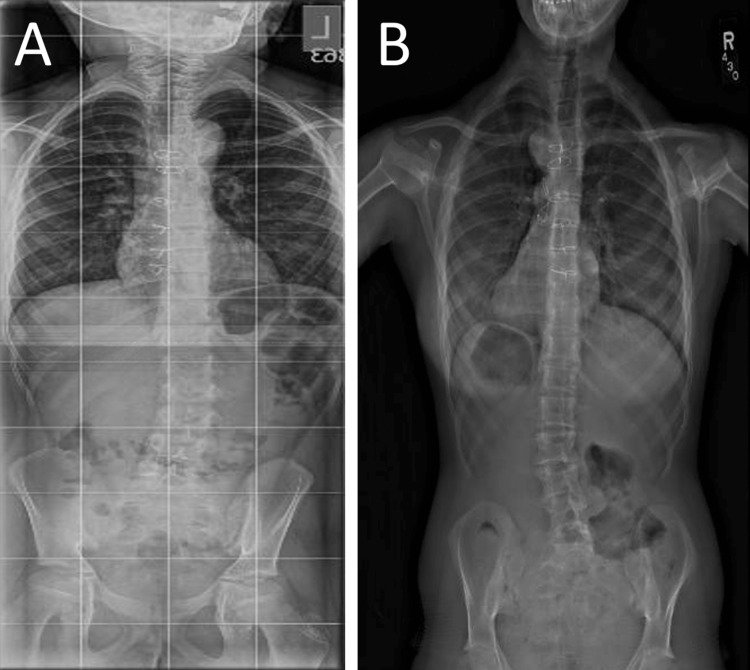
Case one imaging over a five-year period. Initial 36-inch, standing X-ray (A) and most recent X-ray (B) approximately five years apart demonstrating only mild changes to scoliotic pathology. Radiographic imaging obtained over a five-year period demonstrating only mild changes to scoliotic pathology.
